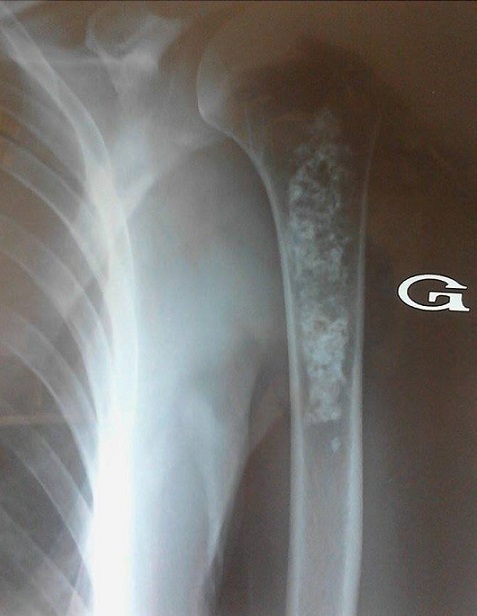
Radiographie du bras: image diaphysaire ostéocondensante irrégulière et en motte du tiers proximal de l'humérus sans effraction de la corticale
